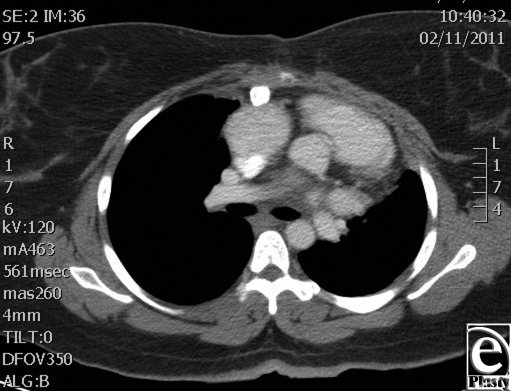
Computed tomographic image shows stable internal reconstruction at 9 months with no fluid collection around the graft.
